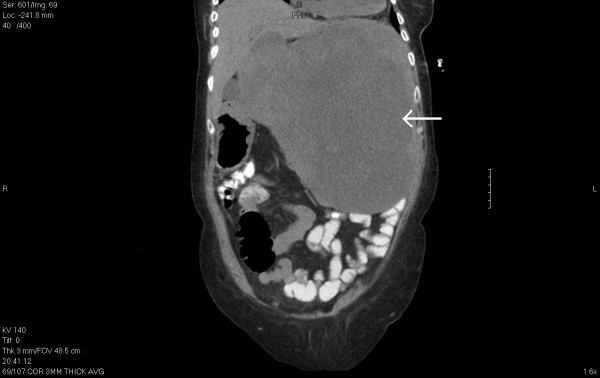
A computed tomographic scan of a large splenic mass (coronal view). See attached arrow.
